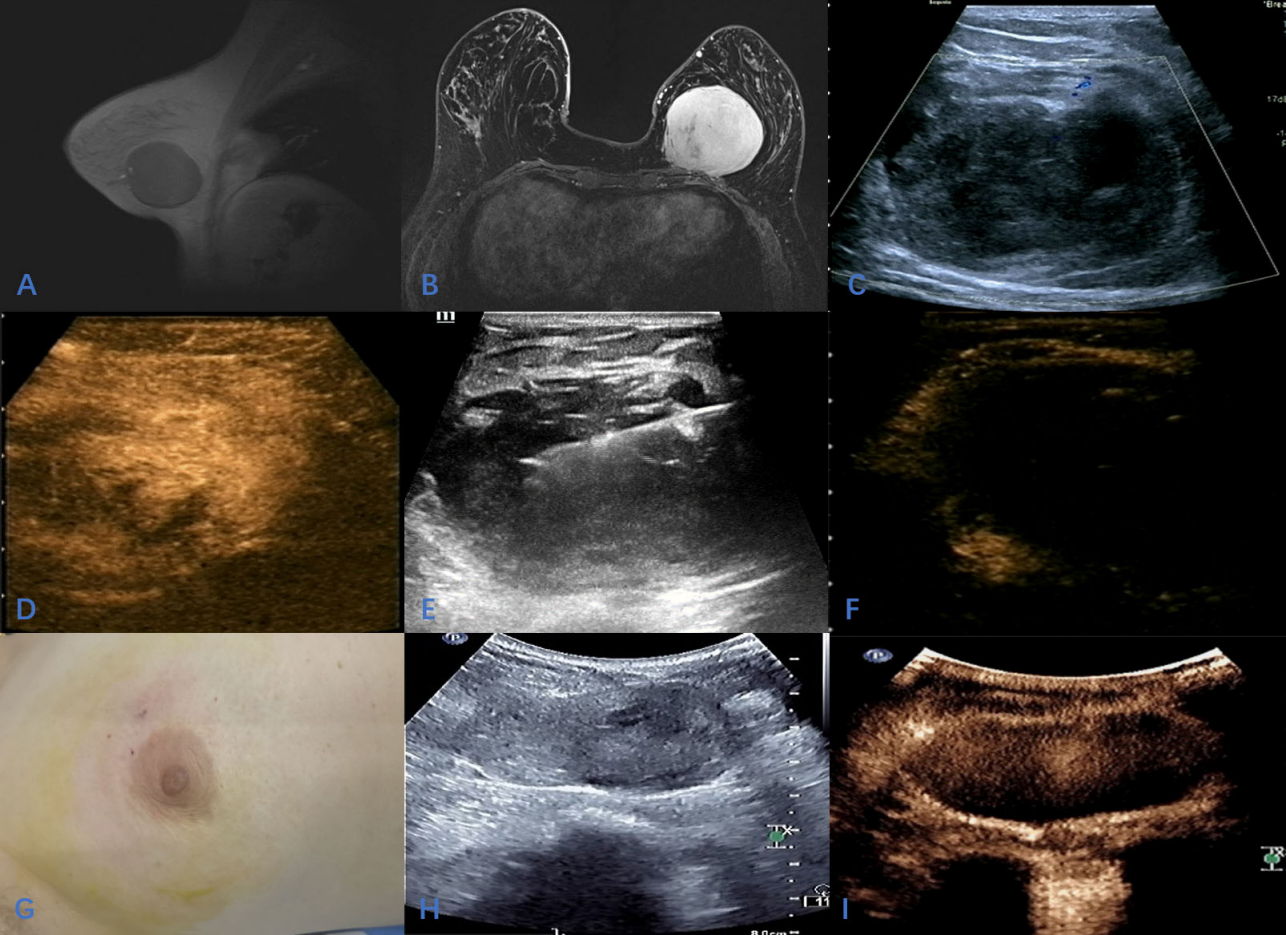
A 37-year-old female with huge mass in the left breast; (A, B) The location of the tumor on enhanced MRI; (C); Sonographic appearance of the tumor on 2D-Ultrasound; (D) Contrast-enhanced ultrasound showed homogeneous hyperenhancement of the tumor during the arterial phase; (E) During ultrasound-guided microwave ablation; (F) The area of ablation showed no enhancement in arterial phase and venous phase after ablation; (G); The skin of the breast was slightly ecchymosis after ablation, and the appearance of the breast was intact; (H, I) After 10 months of follow-up, two-dimensional ultrasound showed that the mass was significantly reduced, and CEUS showed that there was no enhancement in the arterial phase and venous phase of the ablation area.
